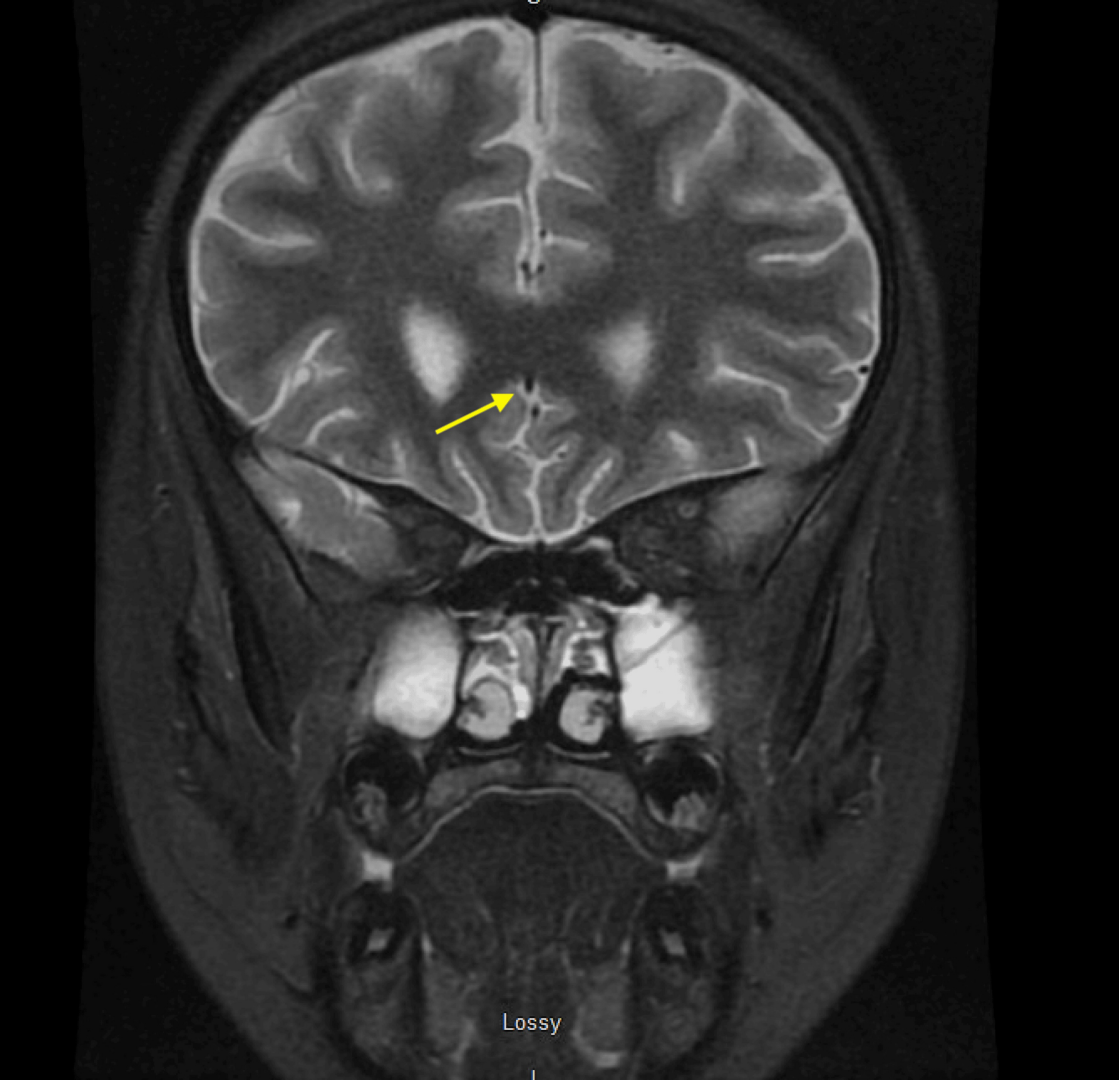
MRI brain w/w/o contrast showing punctate microhemorrhages as well as supra- and infratentorial volume loss MRI: magnetic resonance imaging
